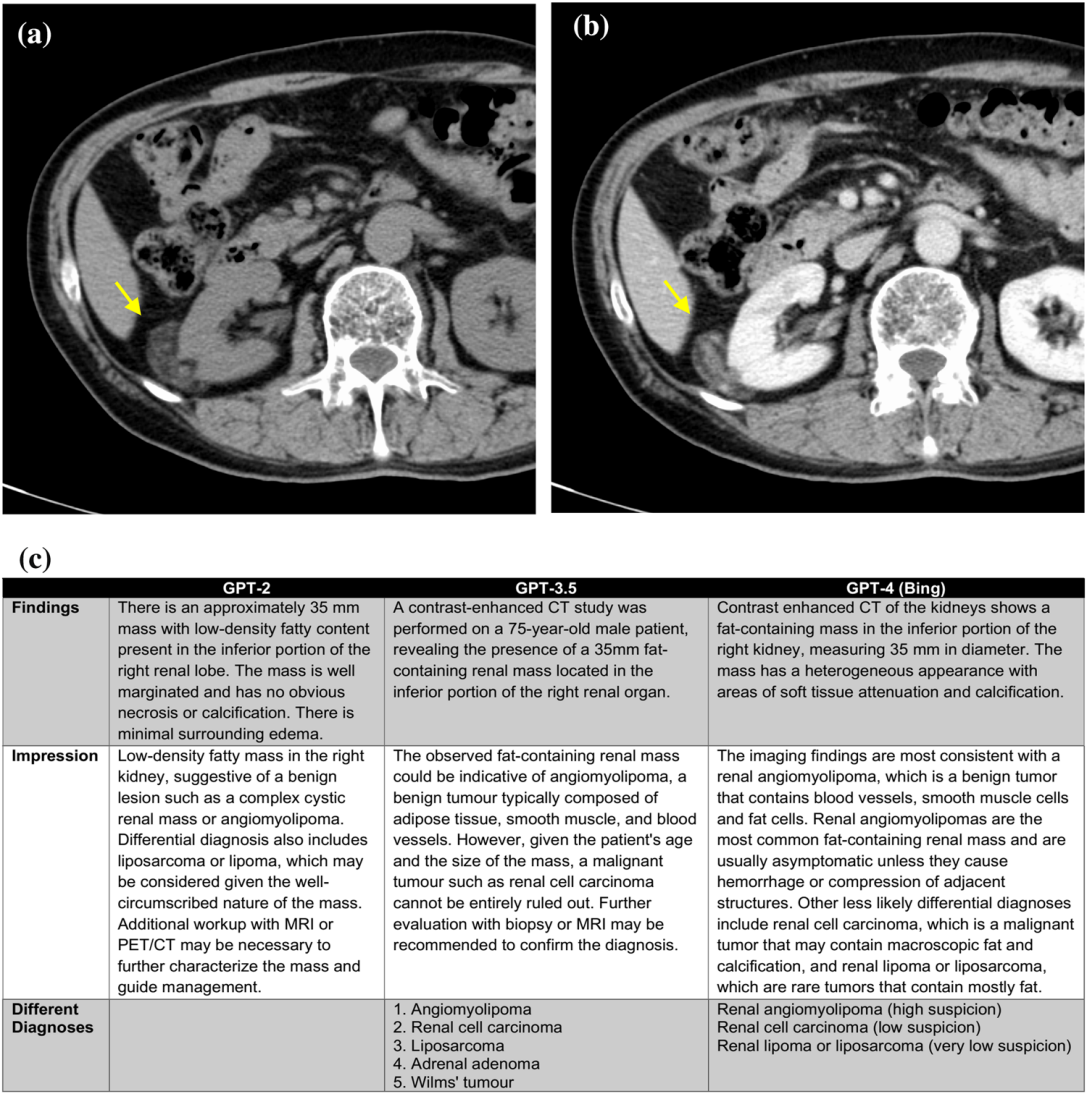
A 75-year-old male with an angiomyolipoma in the right kidney. A non-contrast CT (a) and contrast-enhanced CT (b) reveal a fatty renal mass in the right kidney. Generated radiology reports by GPT series (c) are also shown. The information inputted besides the prompt is “Age (years): 75; sex: male; modality: contrast-enhanced CT; location: the inferior portion of the right kidney; diameter: 35 mm; findings: fat-containing renal mass”. In the GPT-2 generated report, the possibility of a renal tumor accompanied by surrounding edema is low, and a list of differential diagnoses is not even created. In the GPT-3.5 generated report, although the lesion is located in the kidney, the differential diagnoses include adrenal adenoma. In the GPT-4.0 generated report, the overall quality is quite good; however, there is a description of “calcification” in the image findings, which was not part of the input information
